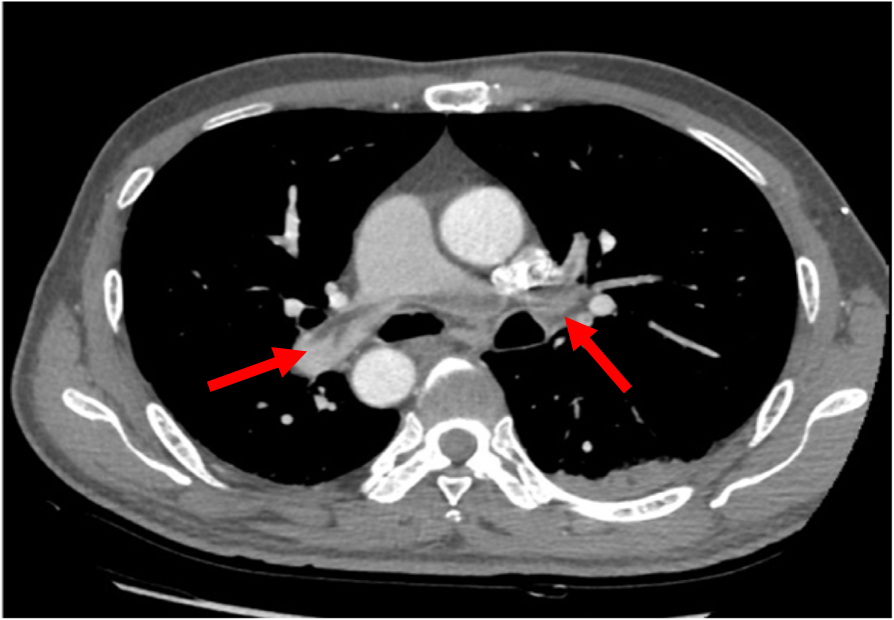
Computed tomography angiogram of the entire aorta showed multiple newly-formed embolus in pulmonary arteries including main branches (indicated by red arrows)
